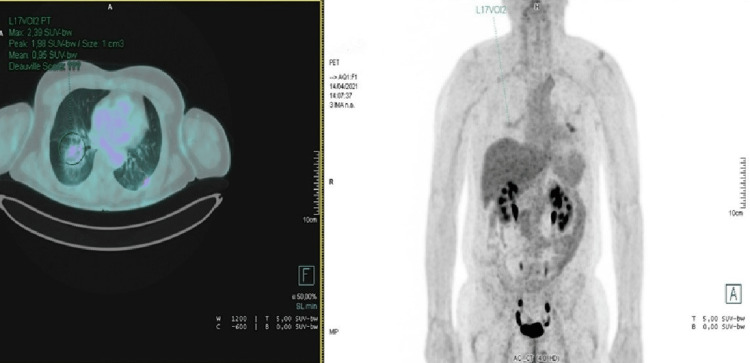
FDG-PET-CT image from April 2021. Right: full body FDG-PET-CT image from April 2021 showing full resolution of previous metastatic lesions. Left: a close-up of the thorax, with no FDG uptake on the right pulmonary lesion. FDG-PET-CT: fluorodeoxyglucose-positron emission tomography-computerized tomography
